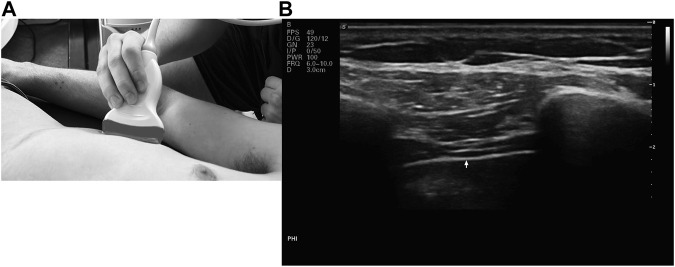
(A) Placement of the linear probe on the rib cage (B) B-mode ultrasound image of the diaphragm.
